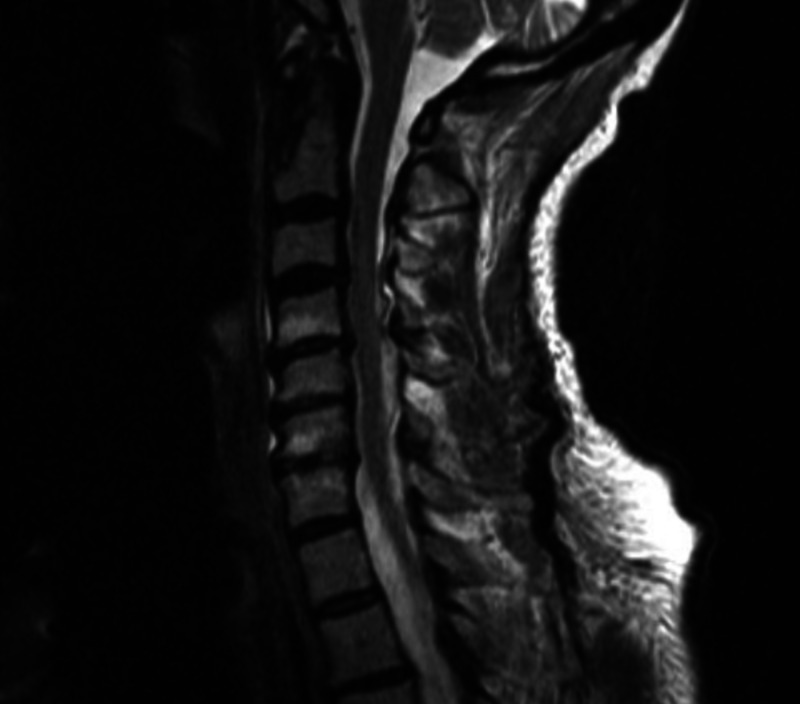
T2 weighted sagittal MRI of the cervical spine reveals moderately severe spondylosis but no kyphosis to explain head drop
